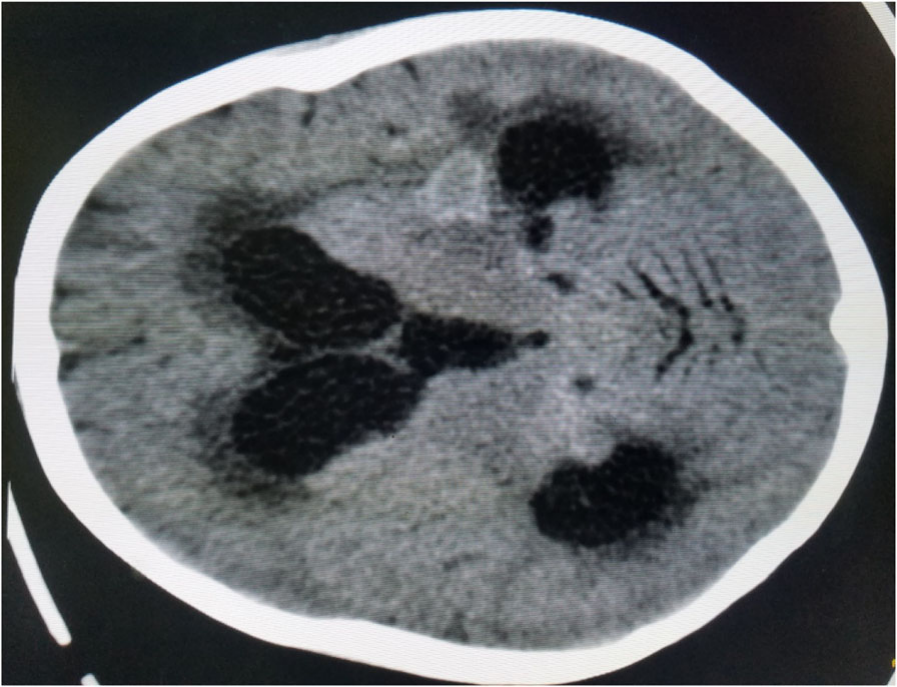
Brain computed tomographic scan showing dilated ventricles with solid tuberculoma
